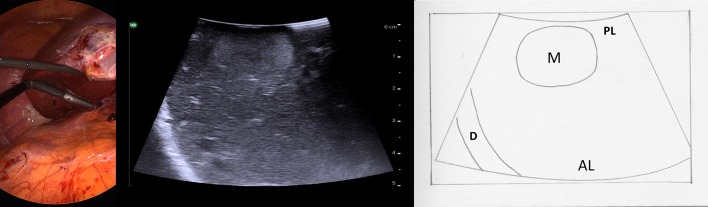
Posterior liver surface contact scanning with the laparoscopic and ultrasound view. M mass, D diaphragm, PL posterior liver, AL anterior liver.
